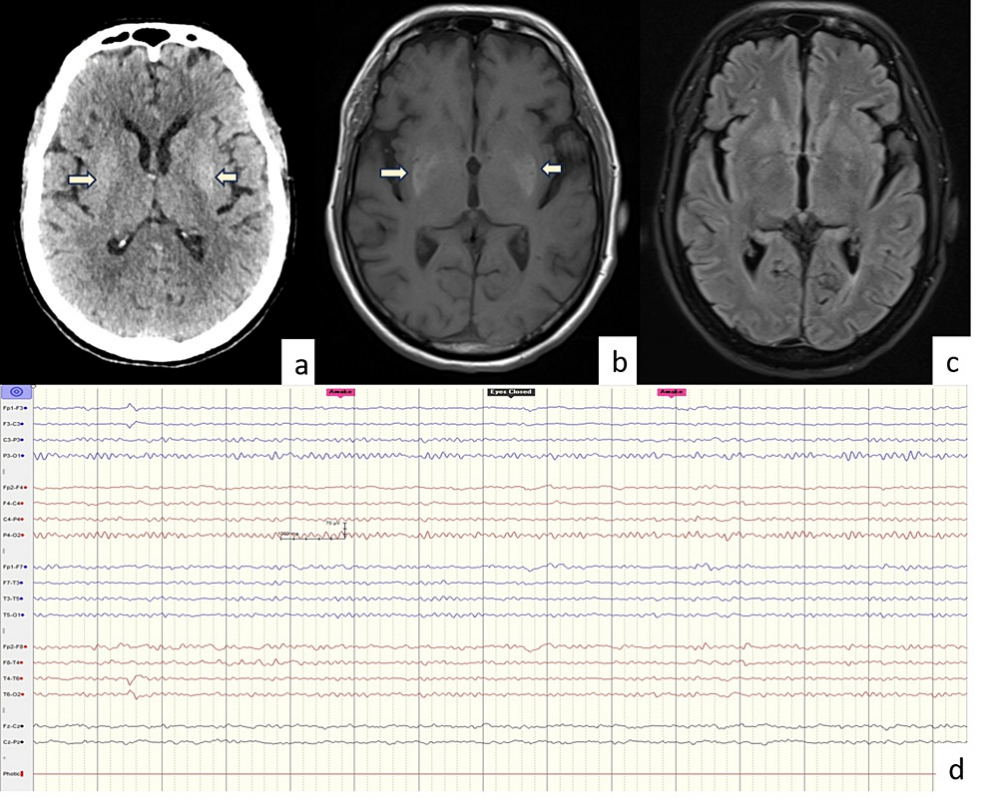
Neuroimaging (a) Brain CT of a 67-year-old male demonstrating hyperdensity (arrow) involving the bilateral lentiform nucleus. (b) MRI (axial) brain of the same patient showing symmetrical hyperintensity (arrow) in the bilateral lentiform nucleus on T1W (b) imaging. (d) Normal EEG of the same patient after the resolution of abnormal limb movements.
